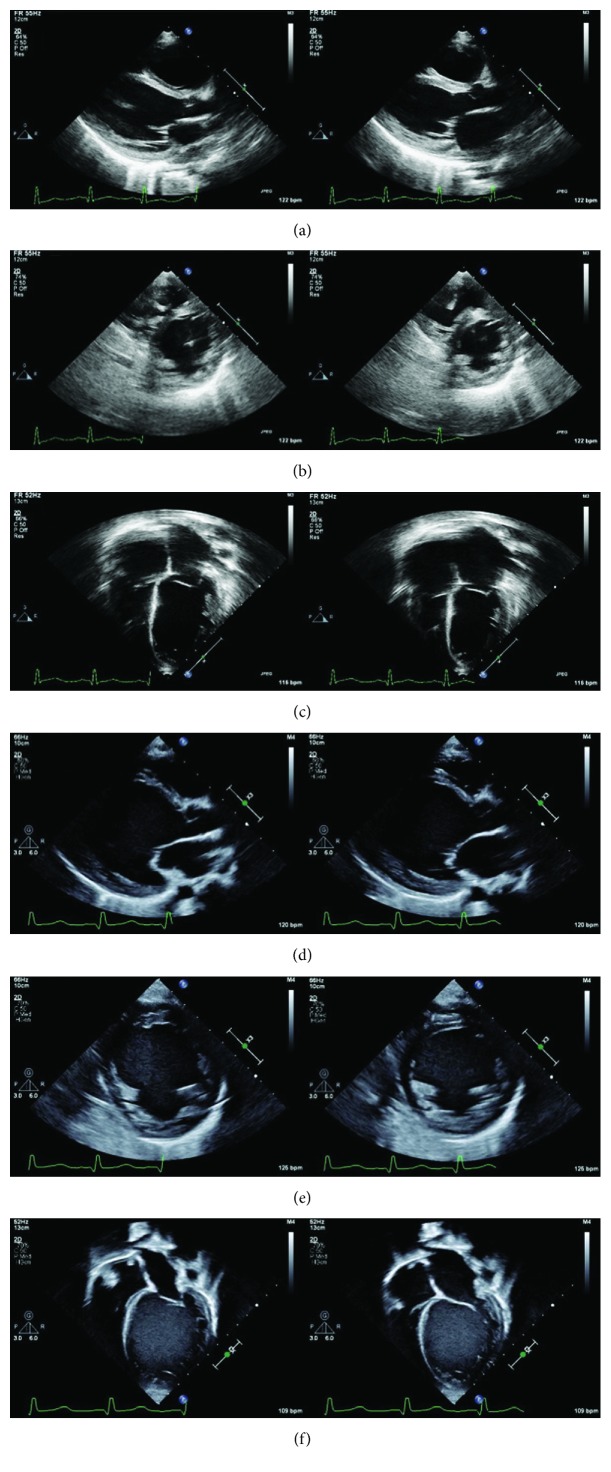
Transthoracic echocardiography (TTE) demonstrating LV size and systolic function at initial presentation (a–c) and 4 months later on milrinone therapy awaiting heart transplantation (d–f). The heart became progressively more dilated and dysfunctional over time. (a) Parasternal long axis view (PLAX) at end-diastole (left) and end-systole (right). (b) Parasternal short axis (PSAX) view at end-diastole (left) and end-systole (right). (c) Apical 4-chamber (A4C) view at end-diastole (left) and end-systole (right). (d) PLAX view at end-diastole (left) and end-systole (right). (e) PSAX view at end-diastole (left) and end-systole (right). (f) A4C view at end-diastole (left) and end-systole (right).
